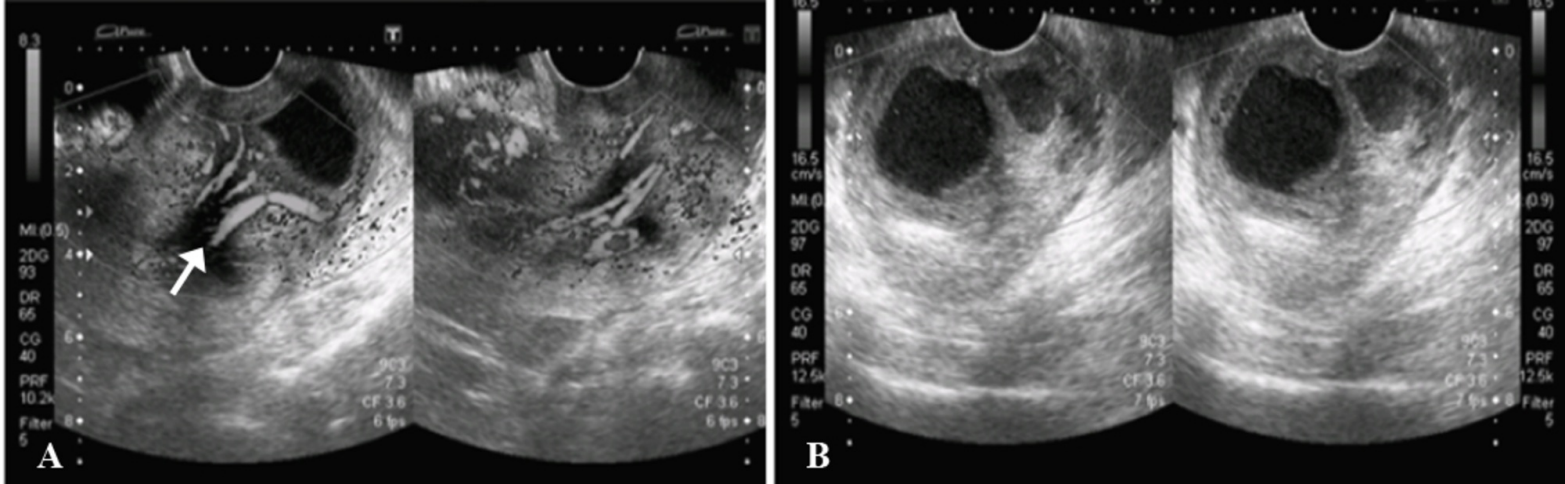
Transvaginal ultrasound images. A) Significant vascularity (arrow) noted on color Doppler in the lower uterine endometrium and cervical canal which was extending into the heterogenous area within the upper vagina. B) Predominantly anechoic cystic lesions are redemonstrated in the vagina, containing fine internal echoes.
